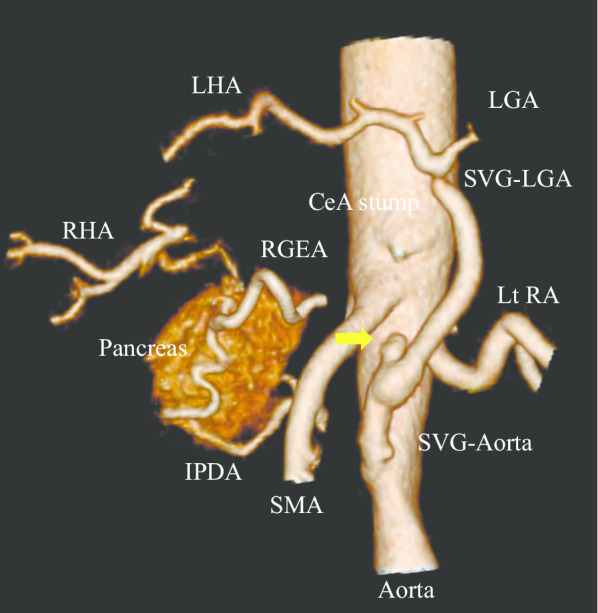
Vascular imaging of postoperative abdominal CT. Yellow arrow indicates the occlusion site of aorta–PHA grafting. Collateral blood flow from the pancreas to the liver was identified. *IPDA* inferior pancreaticoduodenal artery, *RA* renal artery, *RGEA* right gastroepiploic artery
